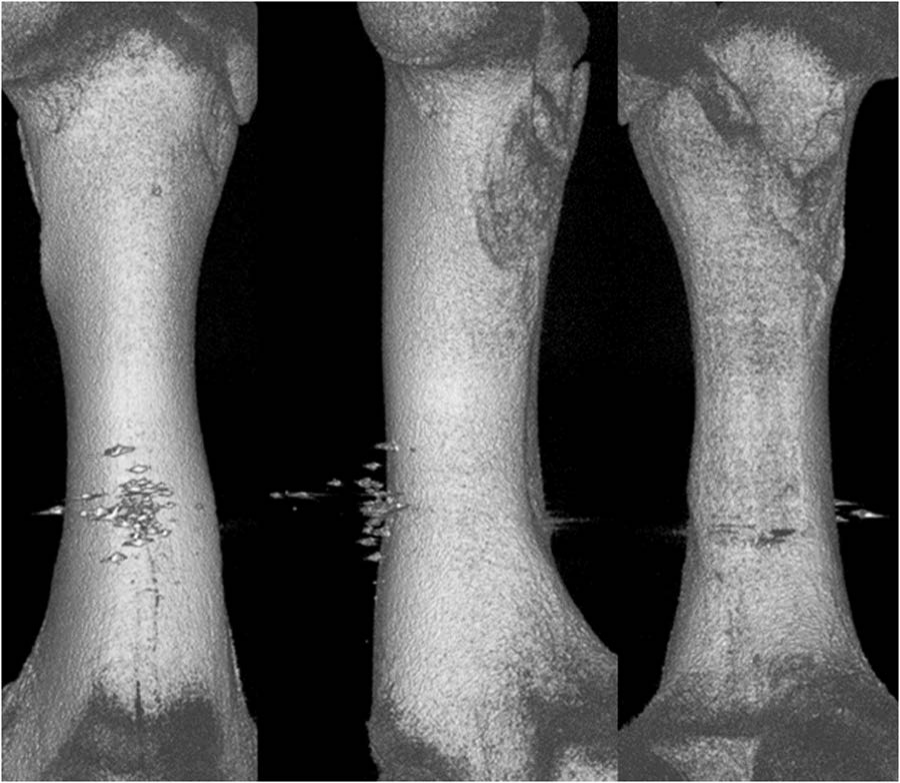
H&N HollowPoint cal. 5.5 mm. 3D CT gunshot damage reconstruction. From the left: anterior, lateral and posterior shaft surfaces.
